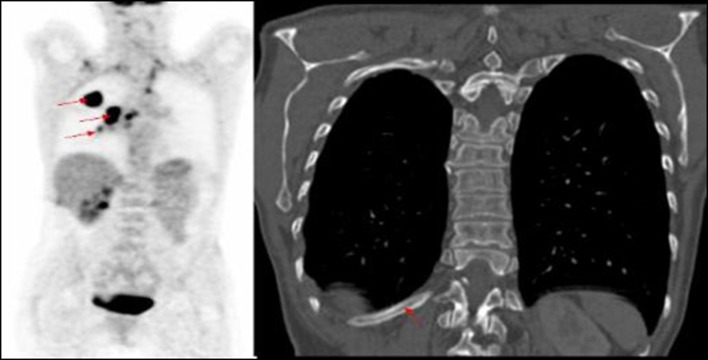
PET image of right lung tumour with right hilar mass and increased uptake in mid thoracic vertebra, not identified on corresponding CT of primary lung cancer.
